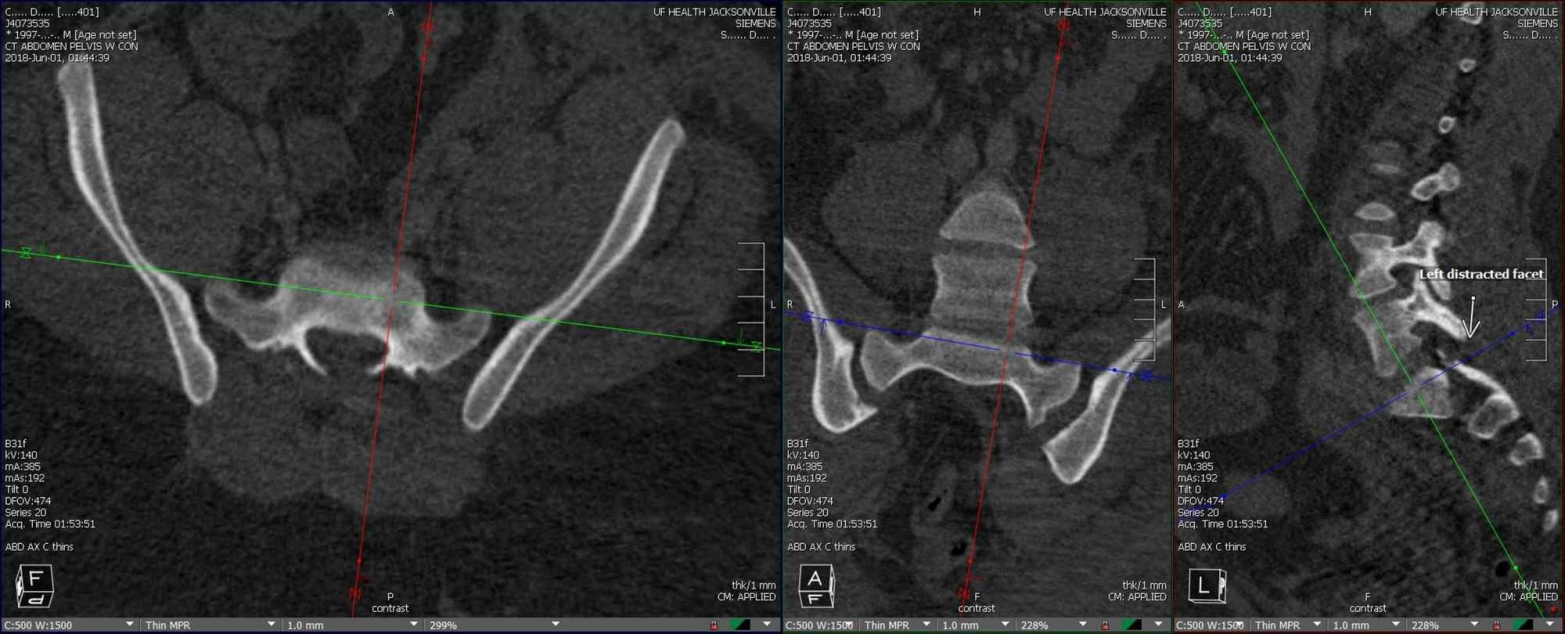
Computed tomography (CT) revealing traumatic lumbosacral dissociation Axial (left) and coronal (center) CT sections revealing sacral pelvic dissociation evident by the widening of the sacroiliac (SI) joints bilaterally. Sagittal (right) CT image revealing the widening of the L5-S1 disc space with posterior listhesis and widening of the L5-S1 facet joints with a fracture facet (not seen on the image).
